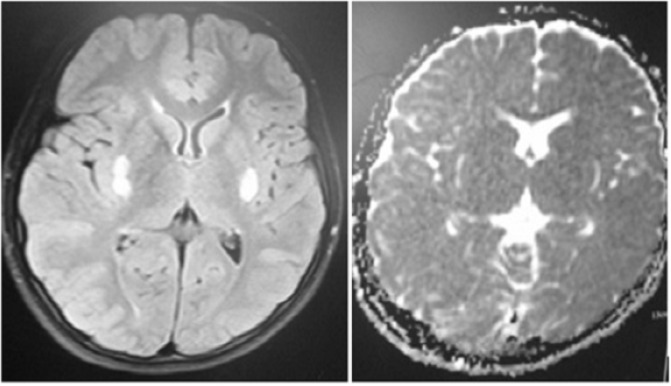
Restriction of the lesions on diffusion weighted (DW) brain magnetic resonance imaging(MRI
